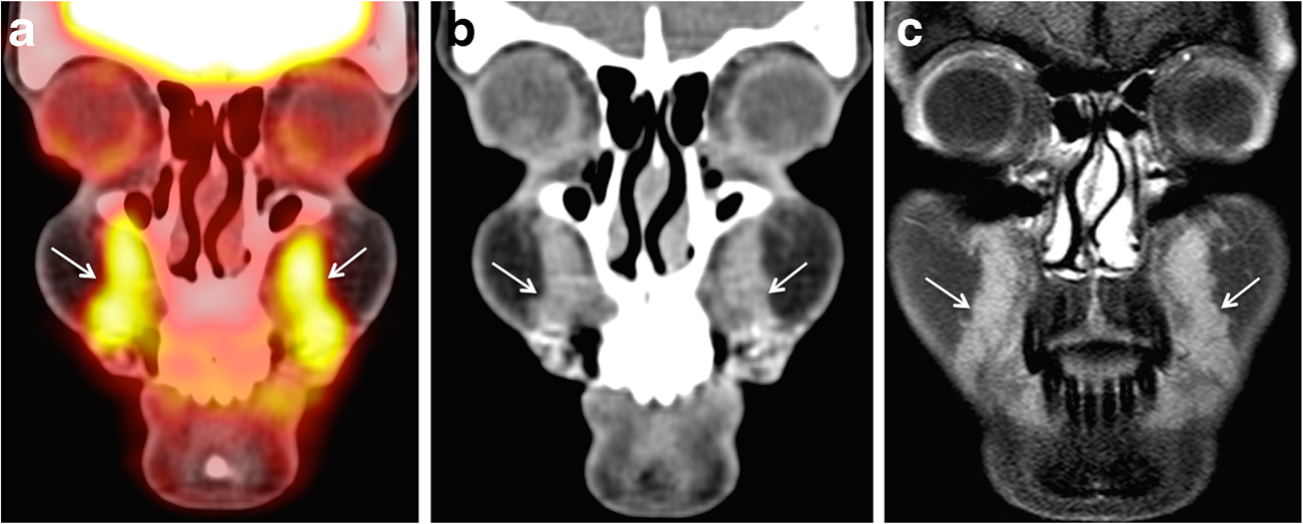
45-year-old female patient evaluated with PET-CT for lymphoma staging. Coronal PET-CT image (a) reveals incidentally detected FDG avid areas in bilateral nasolabial fat compartments (arrows). SUVmean = 4.6, SUVmax = 5.9. The areas appear mildly hyperdense on CT (*arrows* in b) and hardly enhancing on post-gadolinium T1 W fat saturated sequences (c, *arrows*). The patient had a history of silicone injections four years earlier. She had no filler-related symptoms
